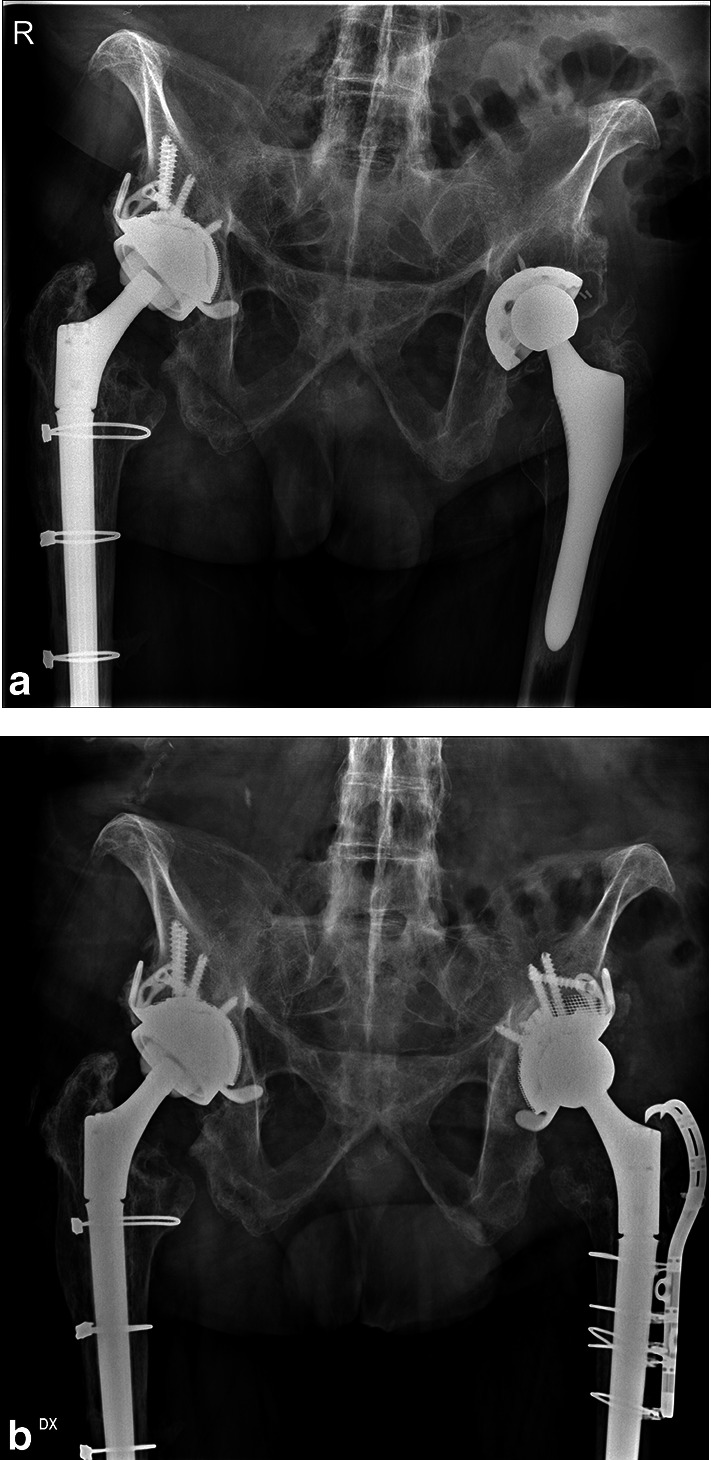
a On right side, Delta TT revision cup at 3 years of follow-up. On left side, preoperative x-rays showed cup loosening and bone defect; b 7 years follow-up on right side and 5 years follow-up on left side both with Delta TT revision cup, and augment and modular conical stems on left side
